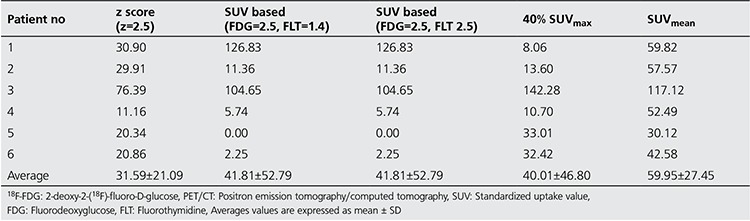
Estimated pancreas tumor volumes using (A) 18F-FDG PET/CT Imaging
